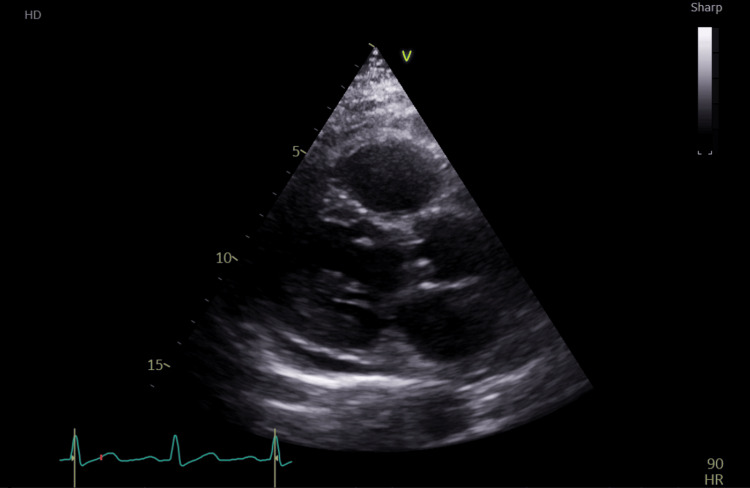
Echocardiography on day 5 of admission shows no evidence of infective endocarditis or vegetations. The LV is of normal size with normal systolic function, with an estimated LVEF of 55%. A small to moderate circumferential pericardial effusion is observed LV: left ventricle, LVEF: left ventricular ejection fraction
